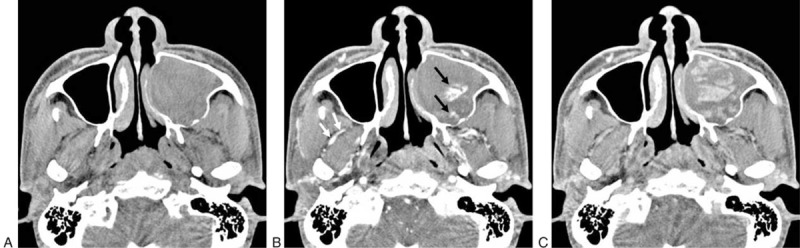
Angiomatous polyp of the maxillary sinus in a 34-year-old man. A: Axial plain CT scan shows that the left maxillary sinus is filled with a mixed-density, uneven expansile mass with remodeling and deformation of the bony wall. B: Early phase axial CT image shows multiple irregular, linear, nodular, and patchy vascular enhancement in the lesion (black arrow), of a similar degree to that of the internal maxillary artery (white arrow). C: Delayed phase axial CT image shows obvious enlargement and fusion of the enhancement region; its degree was still similar to that of the internal maxillary artery. CT = computerized tomography.
